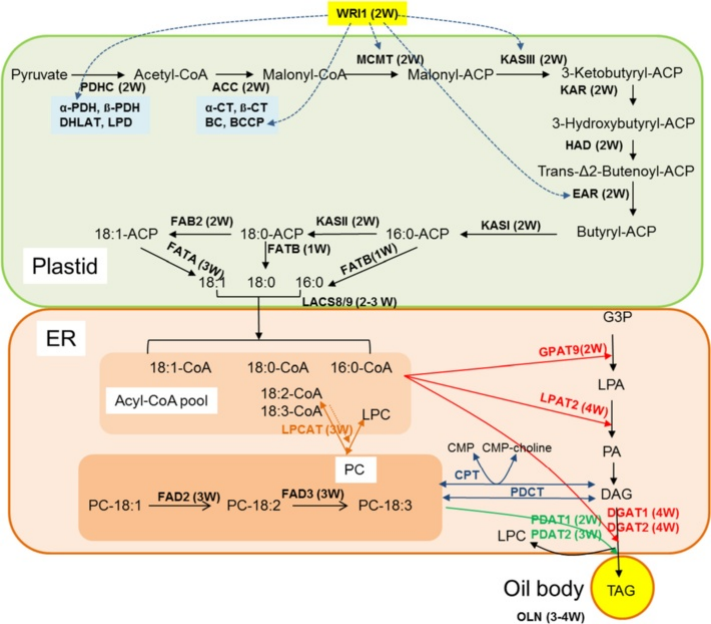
Predicted FA and TAG biosynthetic pathways in perilla *s*eeds. Numbers after the genes (#W) indicate the highest WAF of its expression in developing seeds. Inside of bright green rectangle presents FA biosynthesis and fatty acid export from plastid. Inside of bright red rectangle presents glycerolipids biosynthesis for TAG formation in ER. Yellow circle indicate TAG in oil body. WRI1 transcription factor regulates *ɑ-PDH, BCCP, MCMT, KASIII* and *EAR* genes in FA biosynthesis. Red rectangles inside of ER represent acyl-CoA pools and reaction of desaturation by FAD2 and FAD3 in PC, respectively. Acyl-CoA dependent Kennedy pathway is indicated with red arrow. PC-mediated TAG synthesis pathways are indicated with green (by PDAT), blue (by PDCT and CPT) and orange (by LPCAT). Abbreviations: WRI1, wrinkled1; PDHC, plastidial pyruvate dehydrogenase complex; PDH, pyruvate dehydrogenase; DHLAT, dihydrolipoly acyltransferase; LPD, dihydrolipoamide dehydrogenase; ACC, acetyl-CoA carboxylase; CT, carboxyltransferase; BC, biotin carboxylase; BCCP, biotin carboxyl carrier protein; CoA, coenzyme A; ACP, acyl carrier protein; MCMT, malonly-CoA ACP transferase; KAS, ketoacyl-ACP synthase; KAR, 3-ketoacyl-ACP reductase; HAD, 3-hydroxyacyl-ACP dyhydratase; EAR, 2-enoyl-ACP reductase; FAB2, fatty acid biosynthesis2; FATA, acyl-ACP thioesterase A; FATB, acyl-ACP thioesterase B; LACS, long-chain acyl-CoA synthase; FAD2, Δ12 oleic acid desaturase; FAD3, Δ15 (ω-3) linoleic acid desaturase; GPAT9, glycerol 3-phosphate acyltransferase 9; LPAT2, lysophosphatidic acid acyltransferase 2; LPCAT, lysophosphatidylcholine acyltransferase; DGAT, diacylglycerol acyltransferase; PDAT, phospholipid: diacylglycerol acyltransferase; CPT, CDP-choline:DAG cholinephosphotransferase; PDCT, phosphatidylcholinediacylglycerol cholinephosphotransferase; G3P, glycerol-3-phosphate; LPA, lysophosphatidic acid; PA, phosphatidic acid; LPC, lysophosphatidylcholine; PC, phosphatidylcholine; DAG, diacylglycerol; TAG, triacylglycerol; OLN, oleosin
